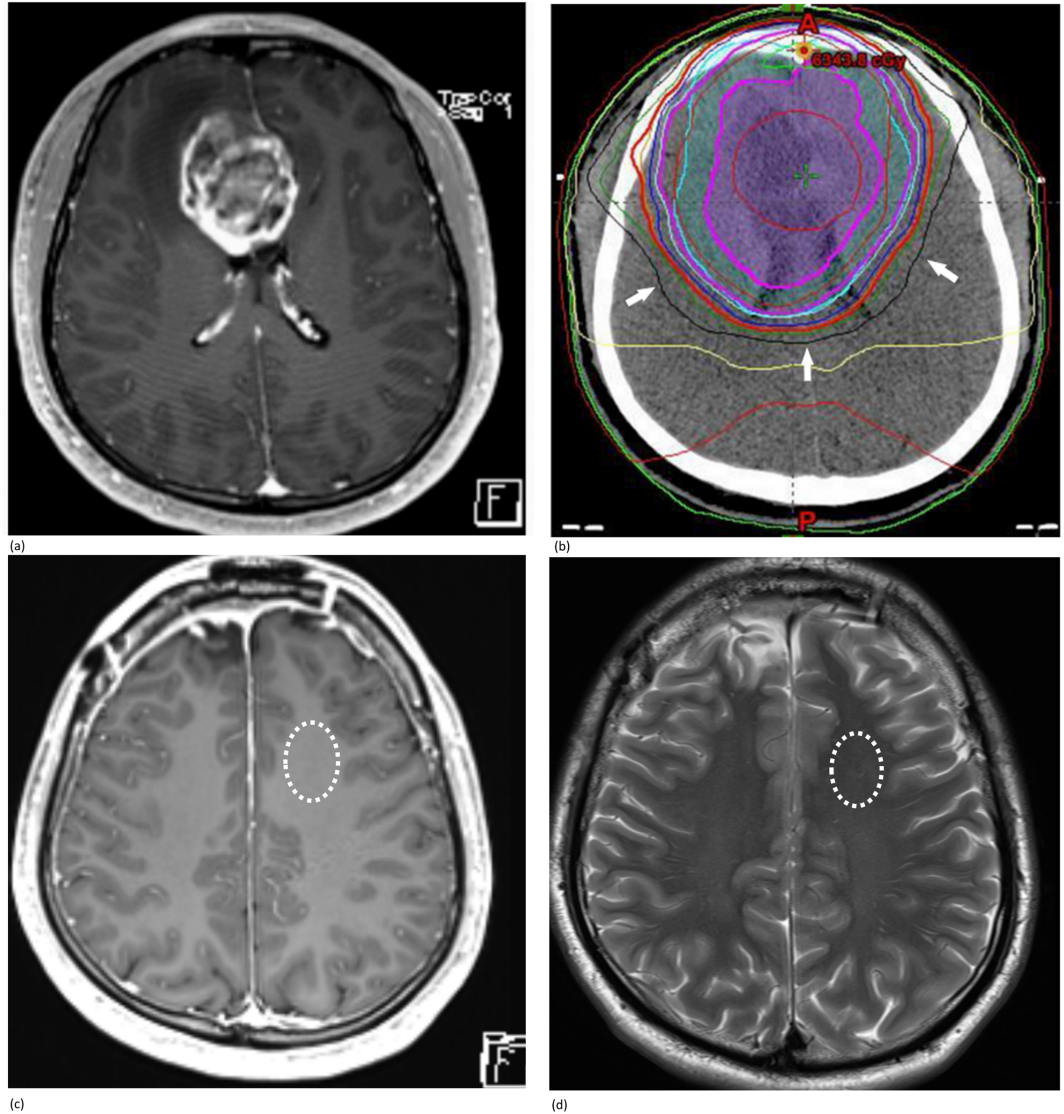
ROI around primary tumors. A 28-year-old male patient with severe headache for 1 month underwent brain MR imaging. (a) Contrast-enhanced axial T1WI revealed a heterogeneous enhancing mass located at the right frontal lobe. He underwent gross total removal of the brain tumor, and final pathology confirmed GBM. He also completed radiotherapy (total dose = 61.2 Gy). (b) The black line (arrows) in the radiotherapy plan map showed the iso-dose line of 3600 cGy, which was the 56.7% iso-dose line of the maximum dose (6343.8 cGy). For R1 measurement, the ROI was drawn at the peri-tumoral area located within the 50–100% iso-dose line of the maximum dose and both (c) overt enhancing area and (d) abnormal T2 hypersignal intensity area were avoided.
